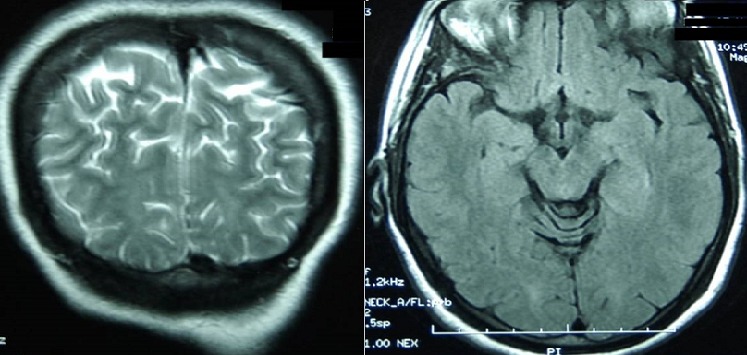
Shows the brain MRI controle did 3 months later with disappearance the cerebral lesions
